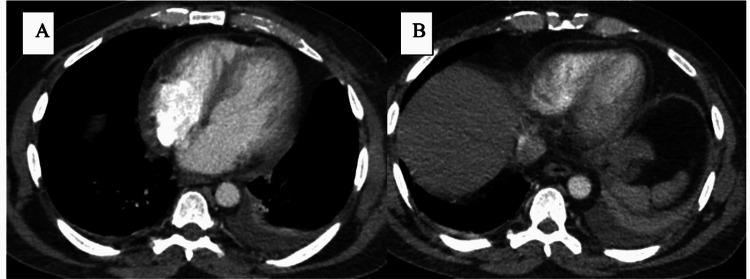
Chest CT showing mild pericardial effusion and left side pleural effusion.
